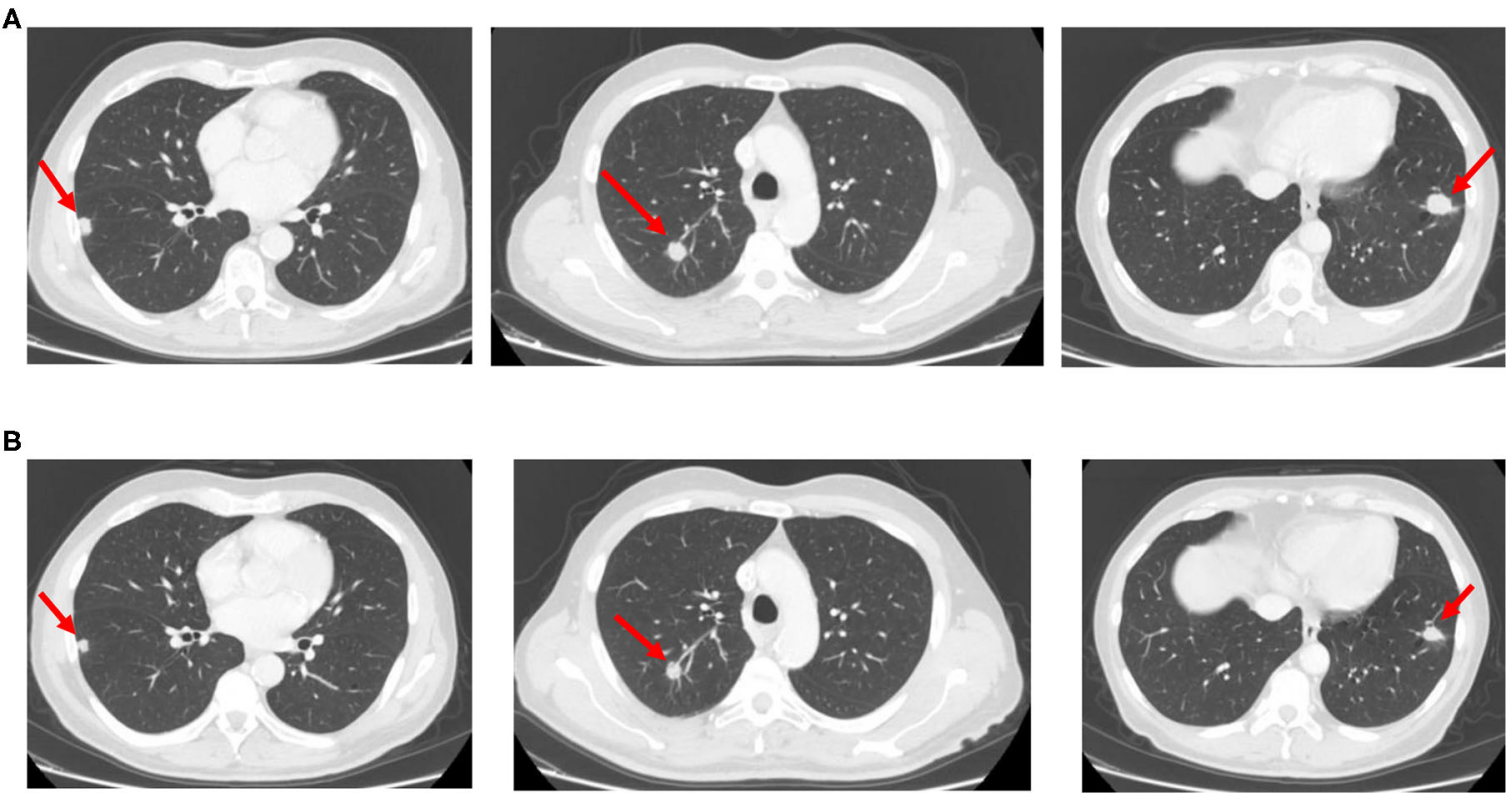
CT scan images showing bilateral lung metastases relapsed after first-line chemotherapy (A) and re-evaluated after aflibercept/chemotherapy re-introduction (B).
